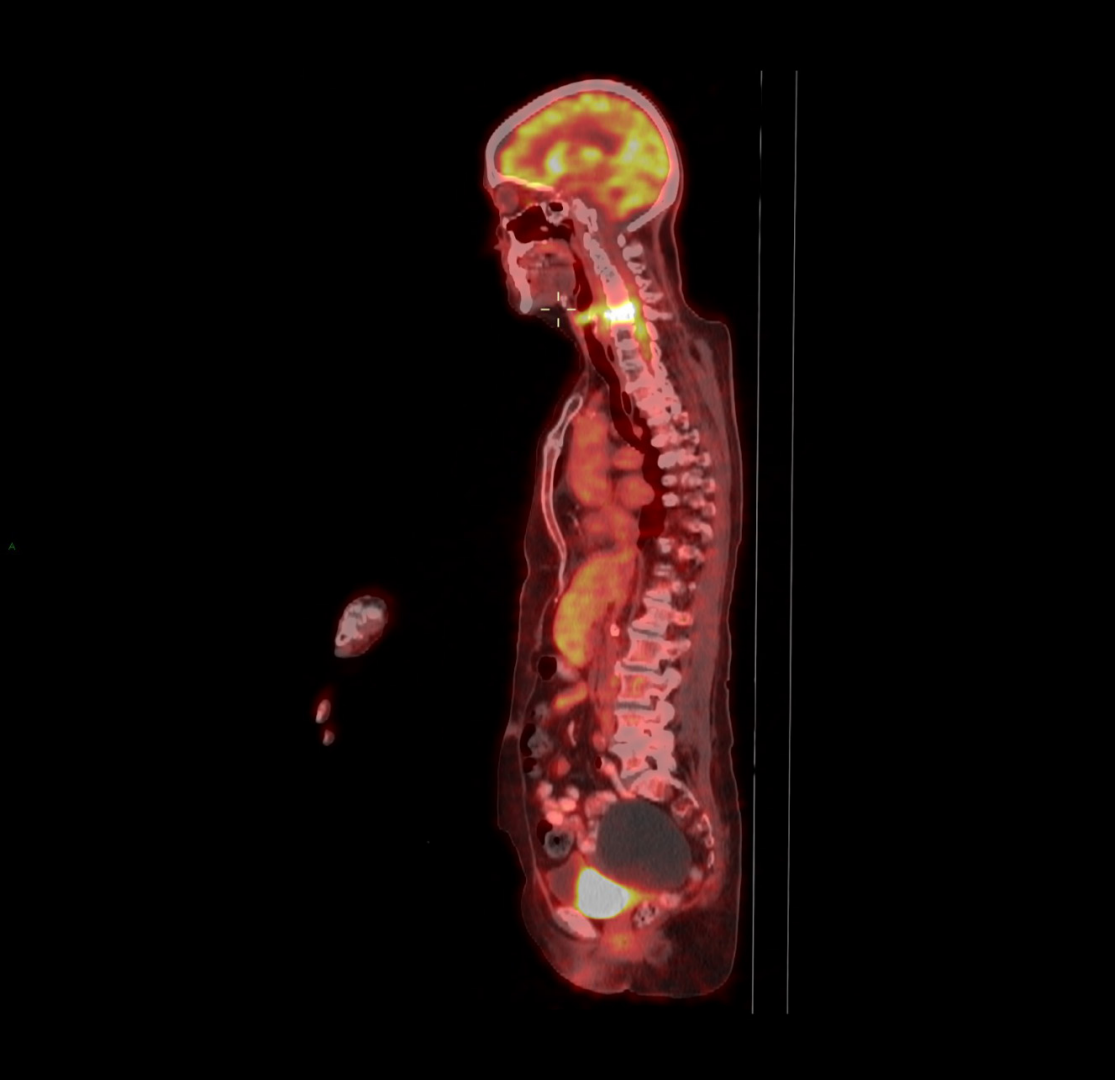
C PET CT show high FDG intake in prevertebral soft tissue and C5-C6 vertebral bodies.
